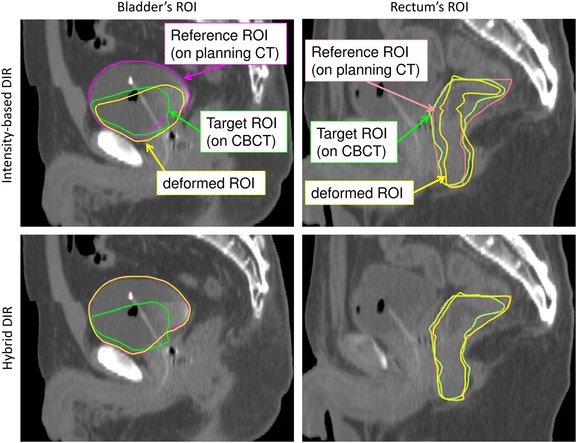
Typical example of rectum and bladder structures based on each DIR (Patient 2). Purple lines: the manual bladder structure on planning CT; green lines: the manual structure on CBCT; yellow lines: the structure deformed by DIR; orange lines: the manual rectum structure on planning CT.
